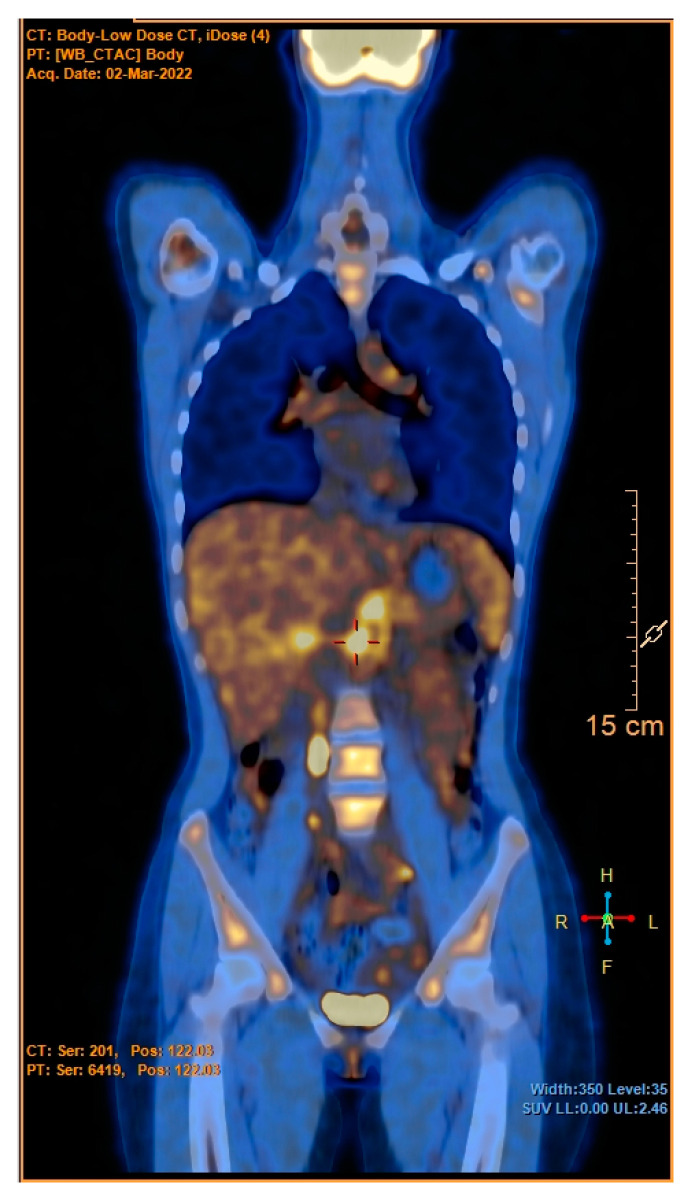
PET-CT. Increased metabolic activity of the suprarenal abdominal aorta.
